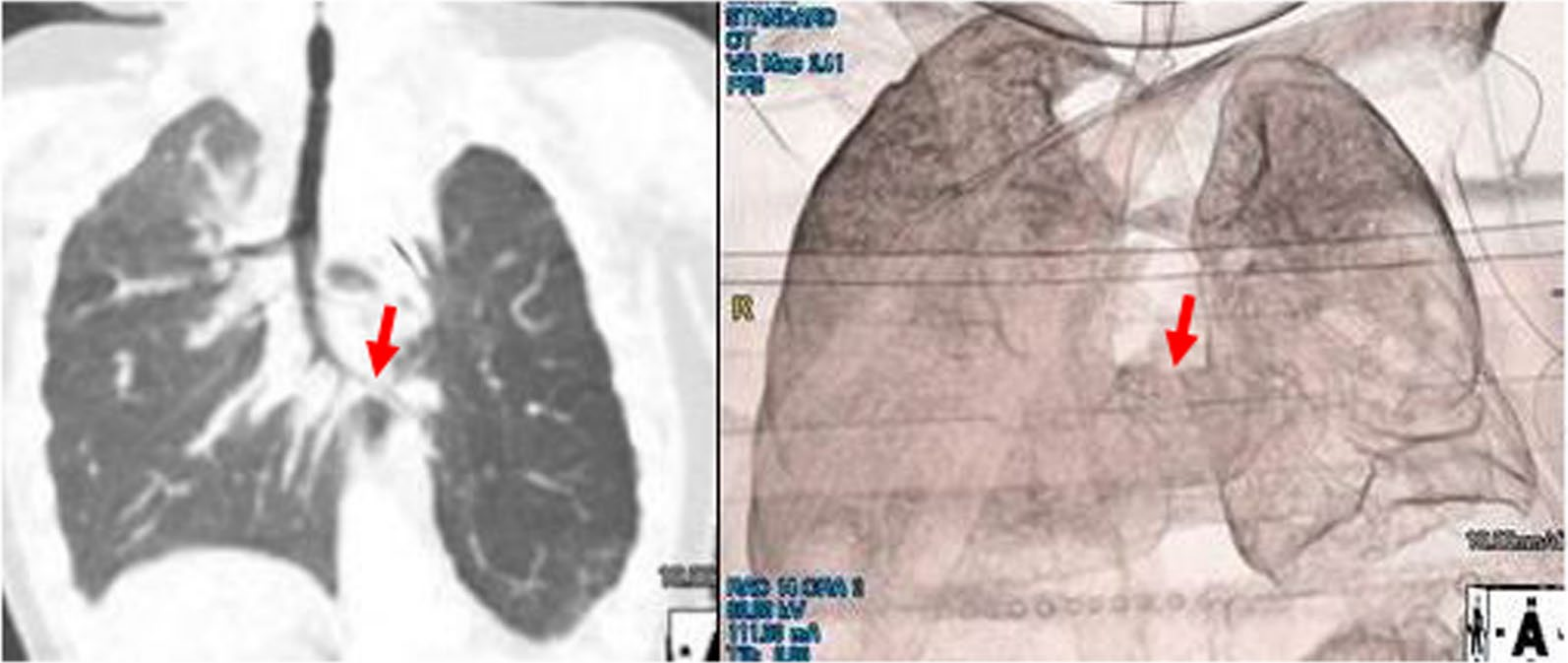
A 4-month-old chest CT image showed no left lung or trachea, and the right lung remained hernia from the dorsal side of the heart into the left thoracic cavity (arrows)
